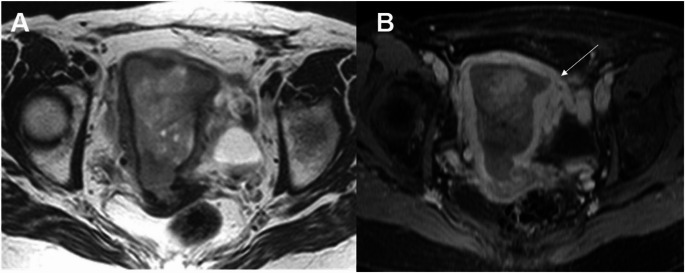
Stage IIC carcinosarcoma, no LVSI in a 67-year-old woman. A Axial oblique T2-weighted fast spin-echo (FSE) image (TR/TE: 4500/100 ms; slice thickness: 3 mm) and B a contrast-enhanced 3D T1-weighted fat-suppressed LAVA sequence (TR/TE: 4.2/2.1 ms; slice thickness: 2 mm; post-gadolinium, delayed phase at 4 min) show a mass invading the superficial myometrium and a peripheral rim of normally enhancing myometrium (arrow)
